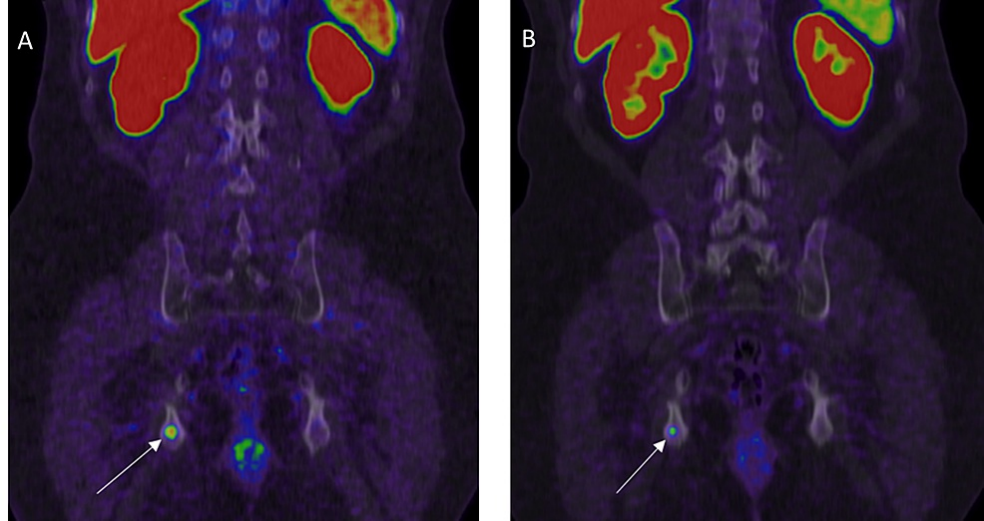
Therapy response A: 68Ga PSMA PET/CT coronal image shows single bone metastasis in the right ischium (white arrow). The lesion was irradiated in this patient on systemic chemotherapy. B: PET/CT six months later shows decreasing uptake of irradiated right ischium metastasis with residual activity denoting viable tumor (white arrow).
